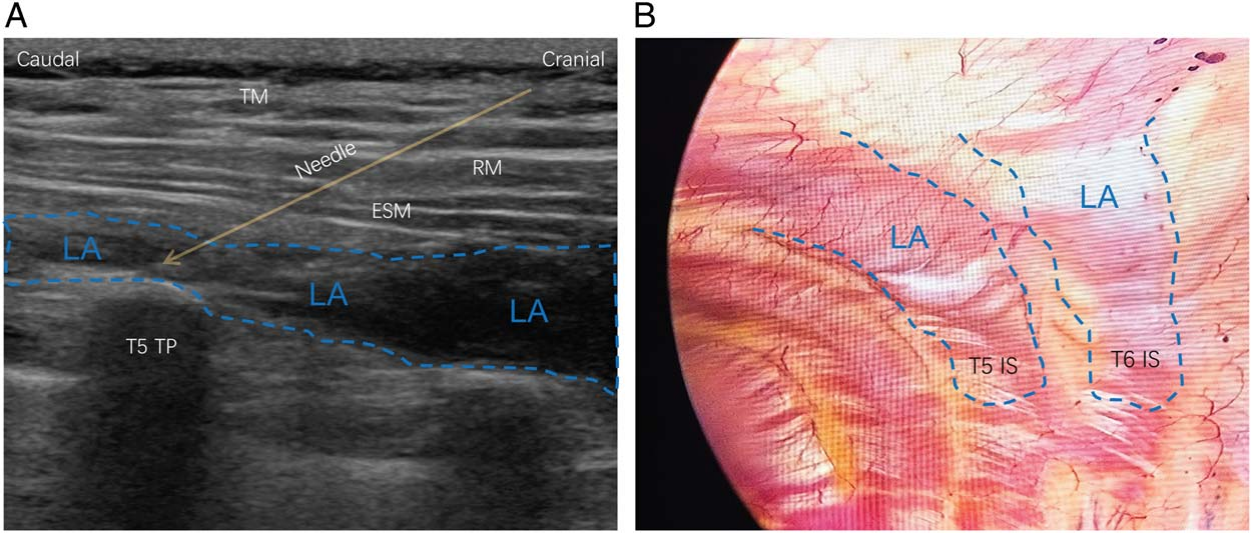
Erector spinae plane block and intercostal nerve block. (A) Ultrasound image of erector spinae plane block. (B) Thoracoscopic visualization of intercostal nerve block. ESM, erector spinae muscle; IS, intercostal space; LA, local anesthetic; RM, rhomboid muscle; TM, trapezius muscle.
